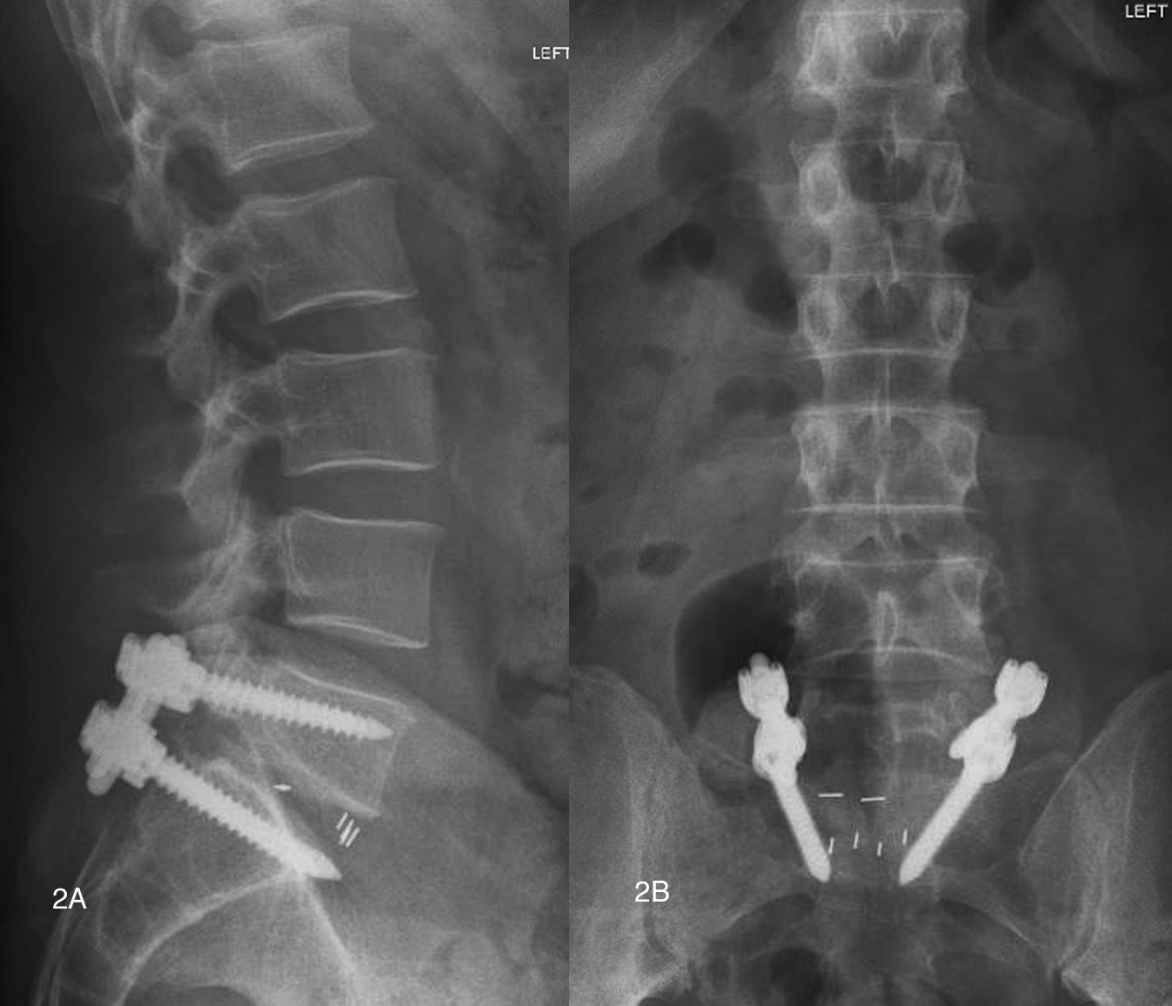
Postoperative lateral and AP radiographs (Figures 2A, 2A) Lateral and anteroposterior radiographs demonstrating appropriate PLIF graft and pedicle screw instrumentation placement.
